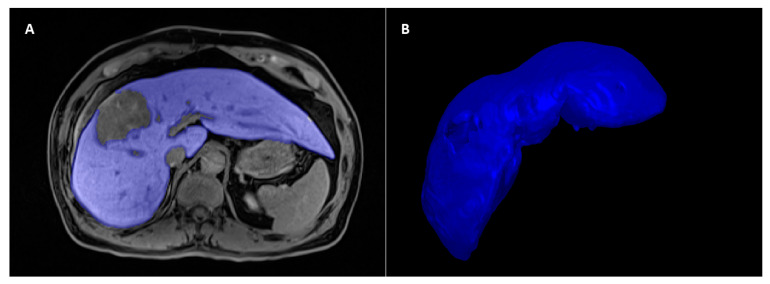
The volume of interest of the non-tumoral hepatic parenchyma was delineated at the hepatobiliary phase image of the preoperative gadoxetic-acid-enhanced MRI (the blue part). (A) Cross-sectional imaging; (B) 3D visualization of the segmented volume of interest.
